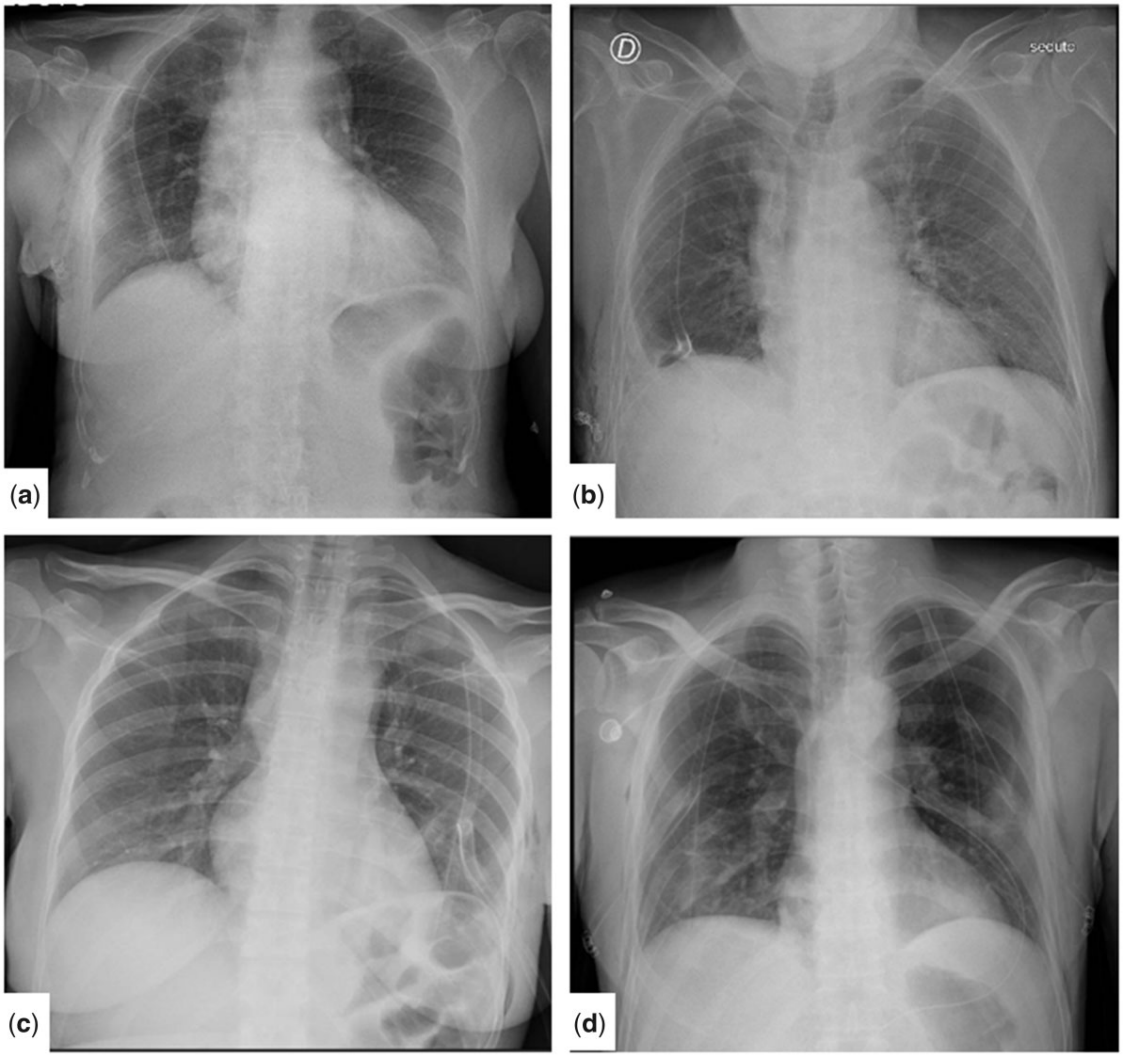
Examples of use of T-tube after uniportal video-assisted thoracic surgery on X-ray. (a) Right wedge resection; (b) right lower lobectomy; (c) left mediastinal biopsy; and (d) bilateral lung metastasectomy (wedge resections).
